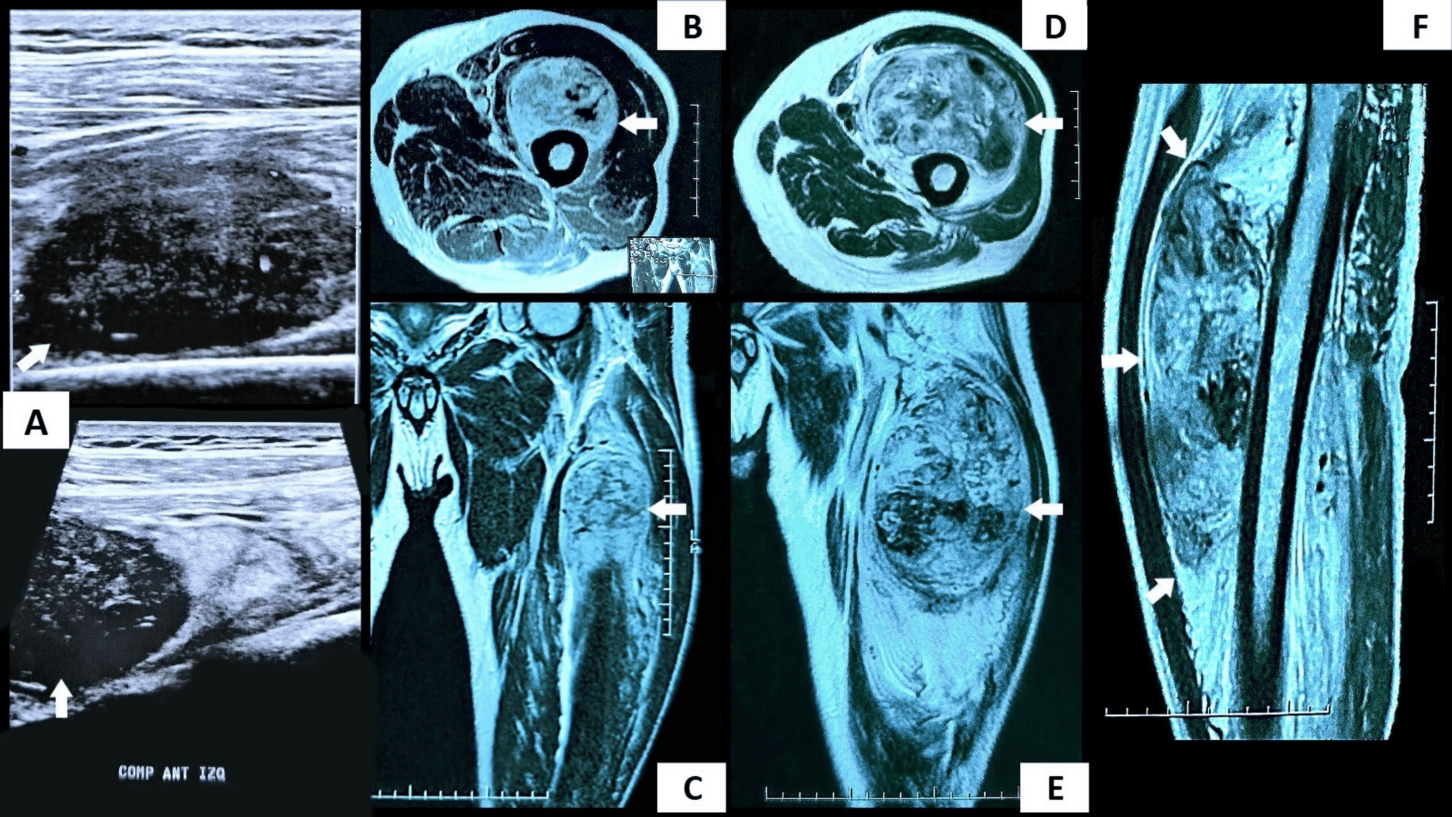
Sequence of images showing accelerated tumor growth during an 86-day period (2.8 months). Cross-sectional view: B and D. Coronal view: C and E. Sagittal view: F. (The tumor is indicated by white arrows). (A) The ultrasound initially shows a mass with dimensions of 2.9 × 4.5 × 5.1 cm (day 1).  (B-C) The MRI shows a mass with dimensions of 3.8 × 5.6 × 10 cm (day 13).  (D, E, and F) The MRI shows a tumor growth of 8.8 × 9.9 × 13.5 cm that persists after neoadjuvant chemotherapy (day 86).
